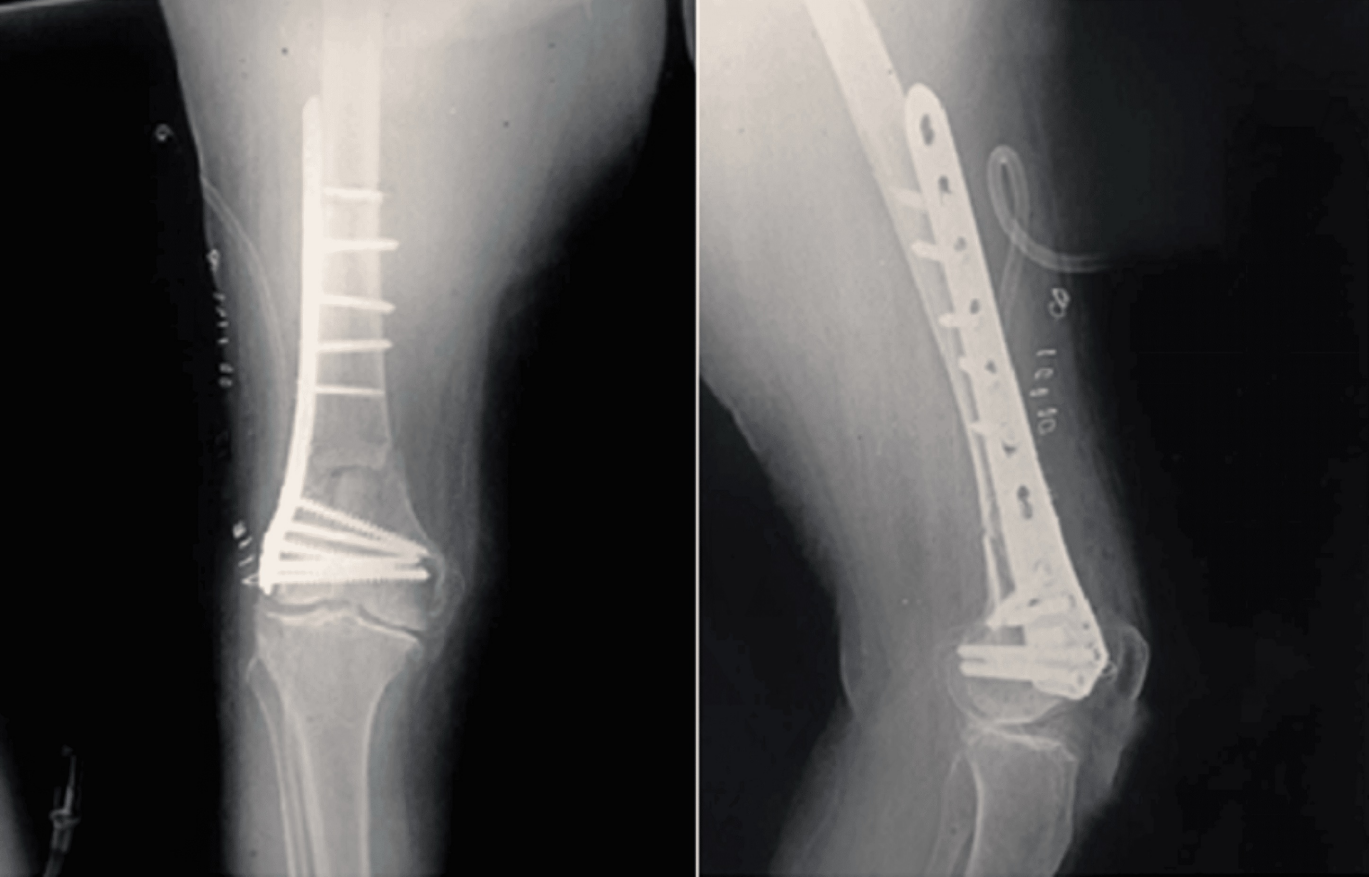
Case 3 immediate postoperative X-rays
